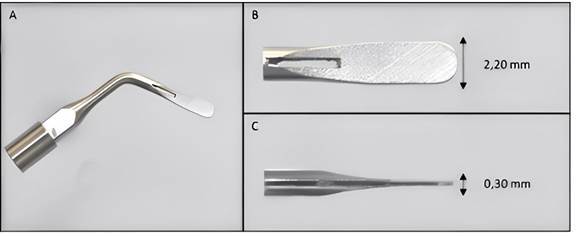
Demonstrative photographic images of the Bladesonic ultrasonic tip (Helse Ultrasonic, Santa Rosa de Viterbo, Brazil). General view of the insert (A), side view of the active part (B) and front view of the active part (C).
